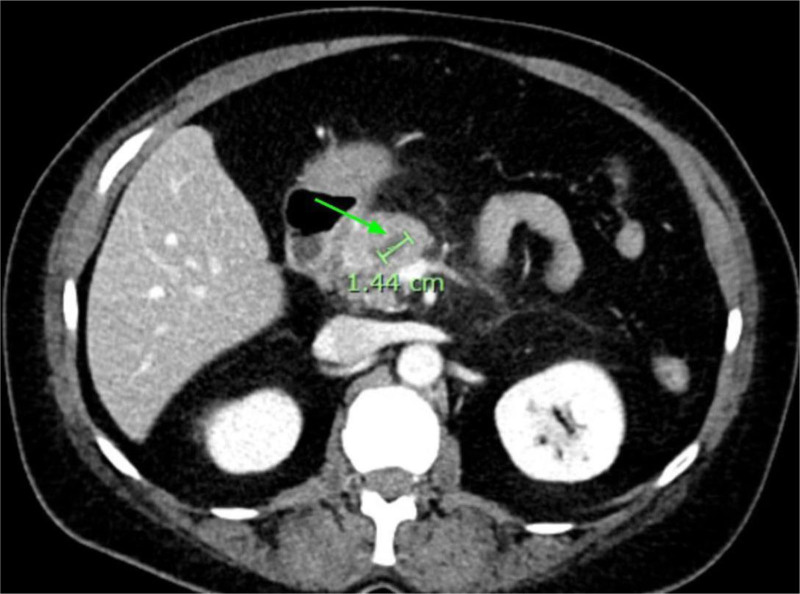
Computed tomography of abdomen and pelvis with contrast. CT revealing a 1.4 cm hypoattenuating focus in the pancreatic neck (green arrow) consistent with either a mass, developing necrosis or focal edematous change.
